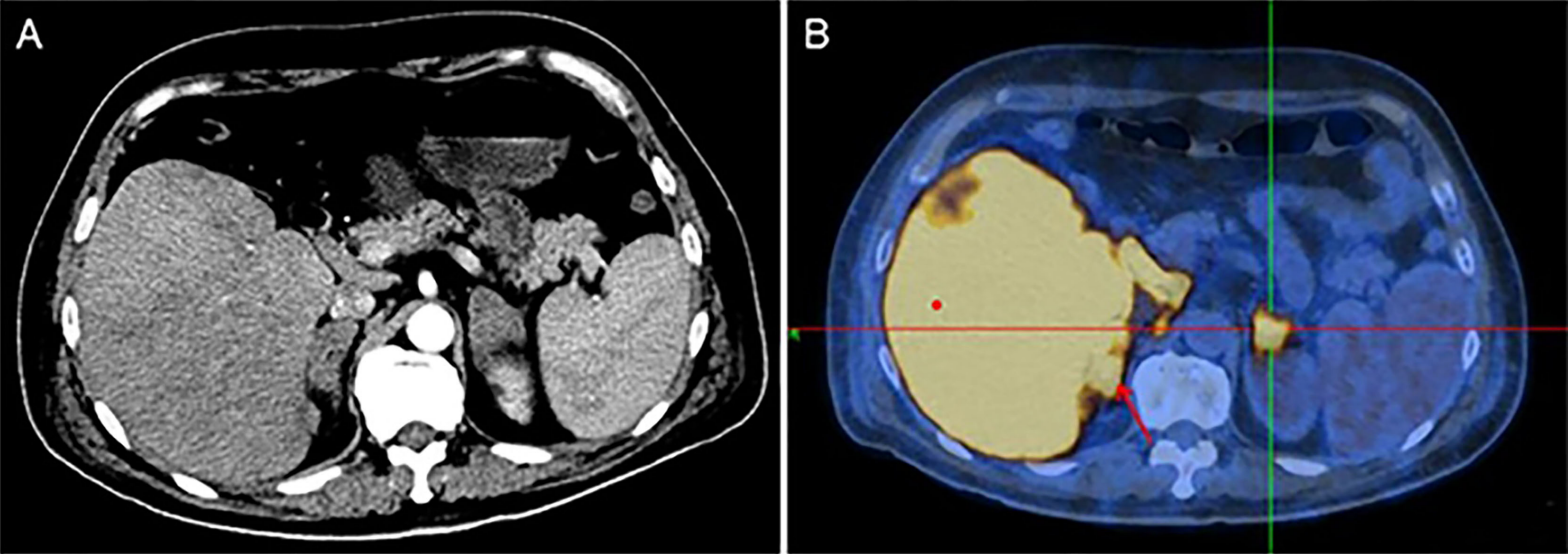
(A) Abdominal CT with contrast enhancement image showed diffuse enlargement of the liver, spleen, and bilateral adrenal glands. (B) PET-CT showed high uptake in liver and bilateral adrenal glands.
